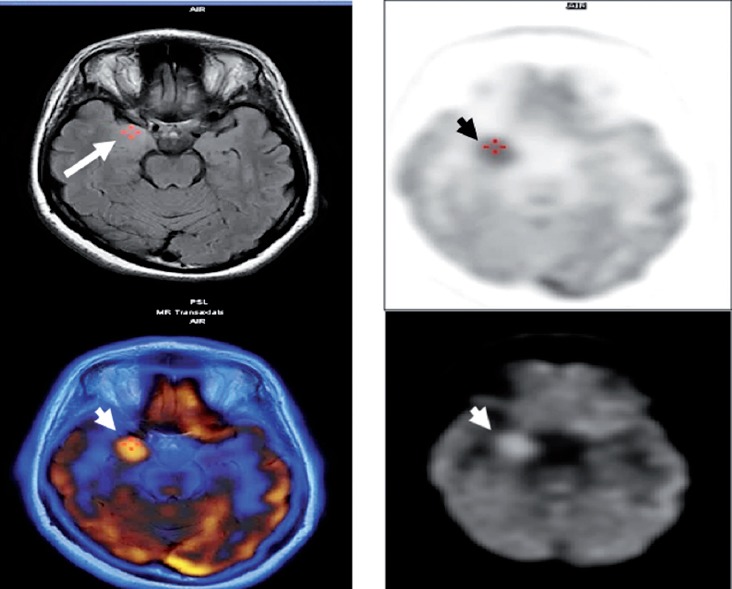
PET scan of the brain at presentation showed hypermetabolic right mesial temporal area (White & black arrow heads) which matched with MRI abnormality (White arrow).
